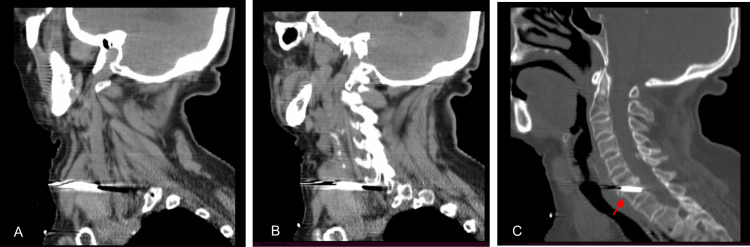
Sagittal enhanced CT reconstructions showing the inferomedial path of the metallic blade piercing the vertebral body of C7 (red arrow)
